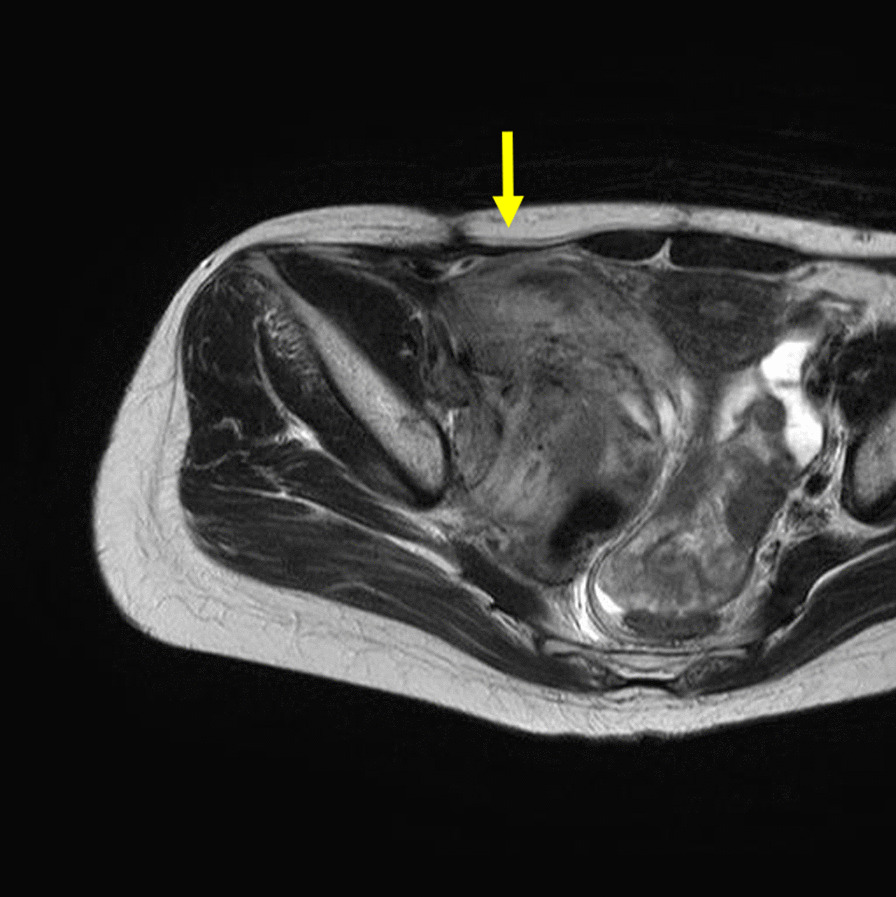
Axial T2-weighted image of the pelvis at 1 month after radiotherapy. Temporary tumor progression is seen (yellow arrow).
